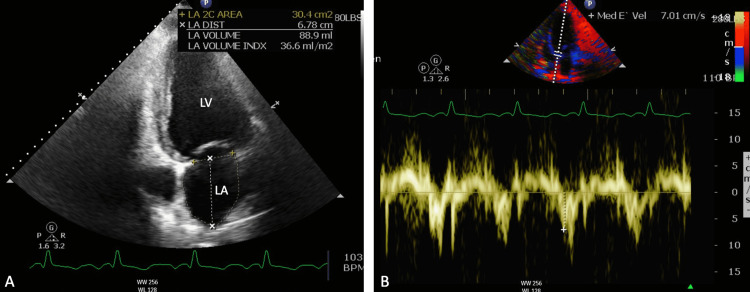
(A) Apical two-chamber view from TTE showing dimensions of LA and LV. (B) Mitral annulus motions from the apical two-chamber view with tissue Doppler imaging showing severe MR. TTE, transthoracic echocardiogram; LA, left ventricle; LV, left ventricle; MR, mitral regurgitation
